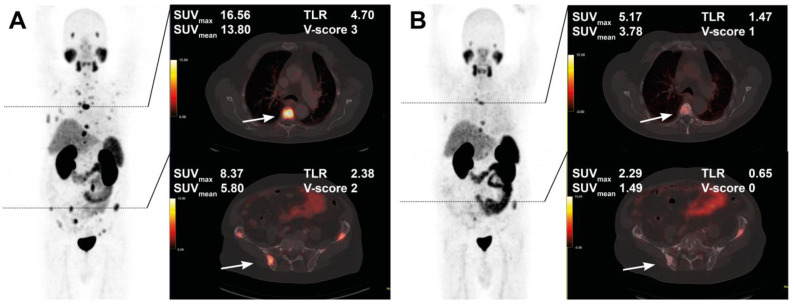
Treatment response in a 76-year-old patient after 4 cycles of RLT with cumulative 32.0 GBq [^177^Lu]Lu-PSMA-617. Maximum intensity projection (MIP) images (left) with cross-section fusion images in a thoracic and pelvic plane at baseline (A) and after RLT (B). Lesions (white arrows) show response to RLT, with the upper lesion in the thoracic spine maintaining detectable residual activity.
